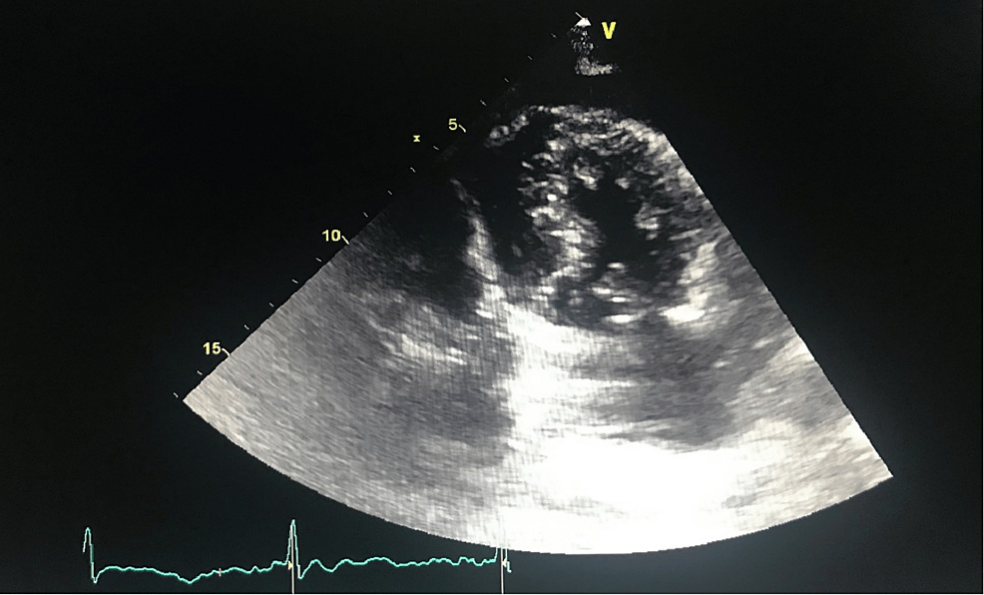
Echocardiogram (apical view) showing evidence of large pericardial effusion with diastolic collapse of the right ventricle
